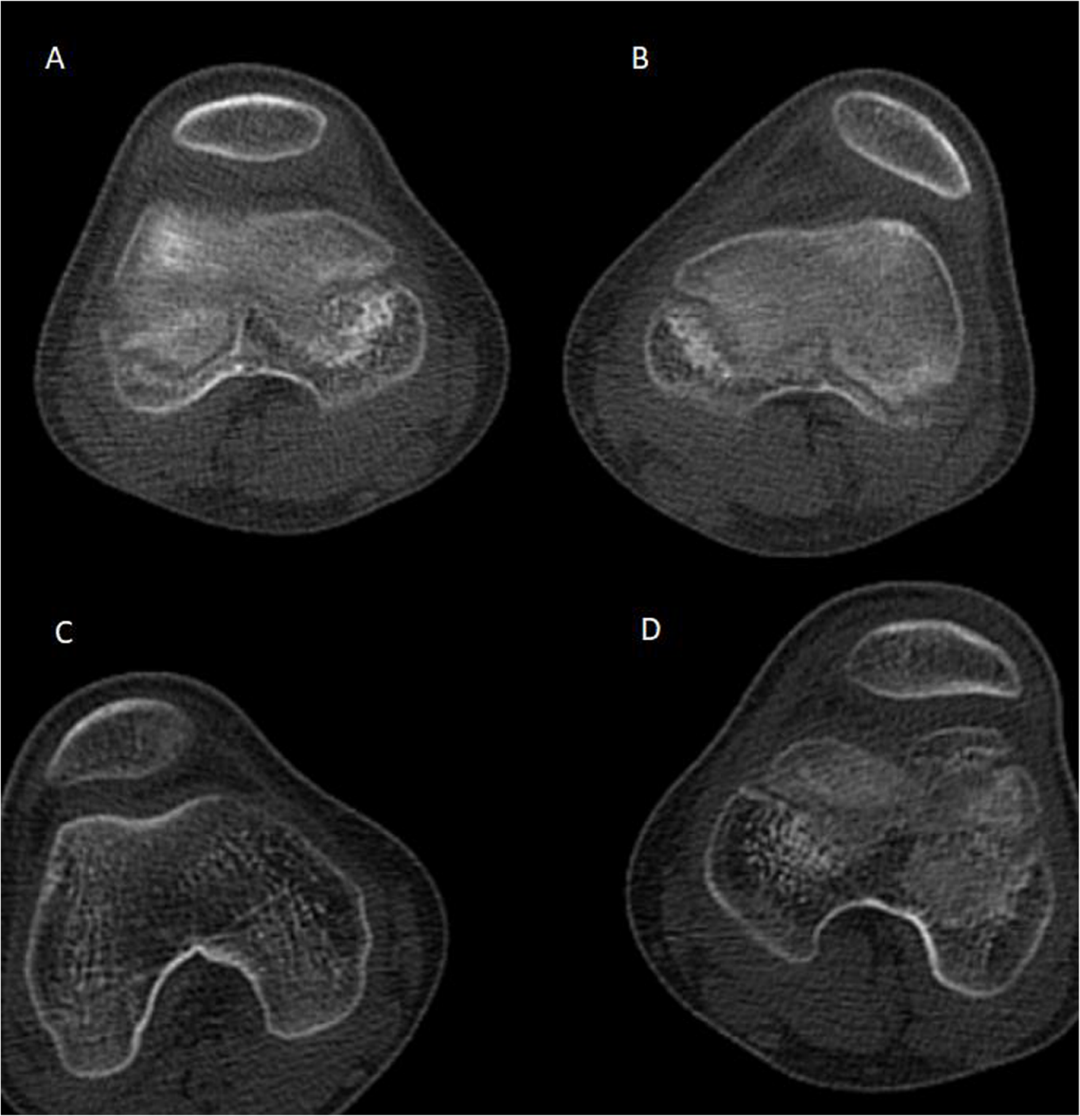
The axial CT of the patella before surgery and at the last follow-up in one patient. Patella A is in the unaffected side and Wiberg-index is 0.61. Patella B is in the affected side and the Wiberg-index is 0.78. Patella C develops from patella A at the last follow-up and the Wiberg-index is 0.64. Patella D develops from patella B at the last follow-up and the Wiberg-index is 0.69
